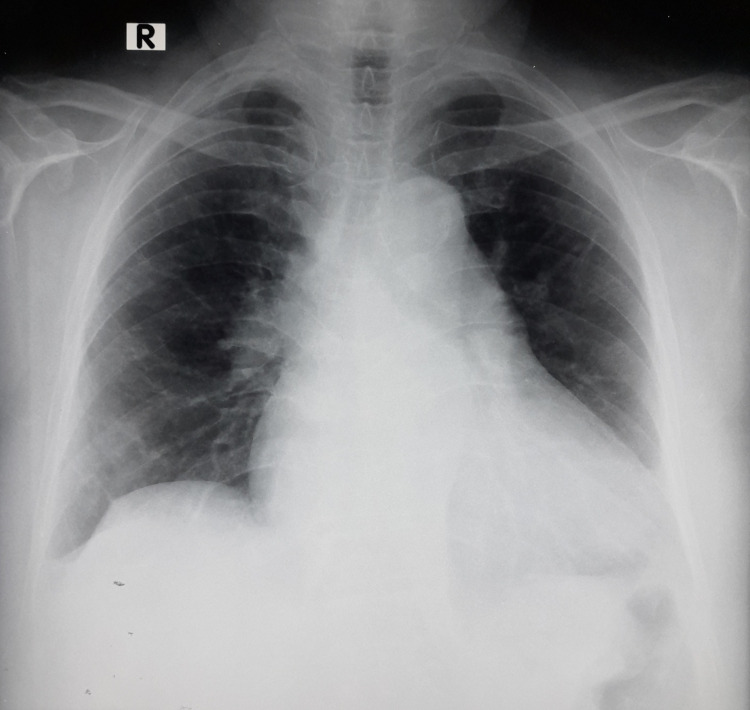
Chest X-ray (PA) demonstrating cardiomegaly PA: posteroanterior
